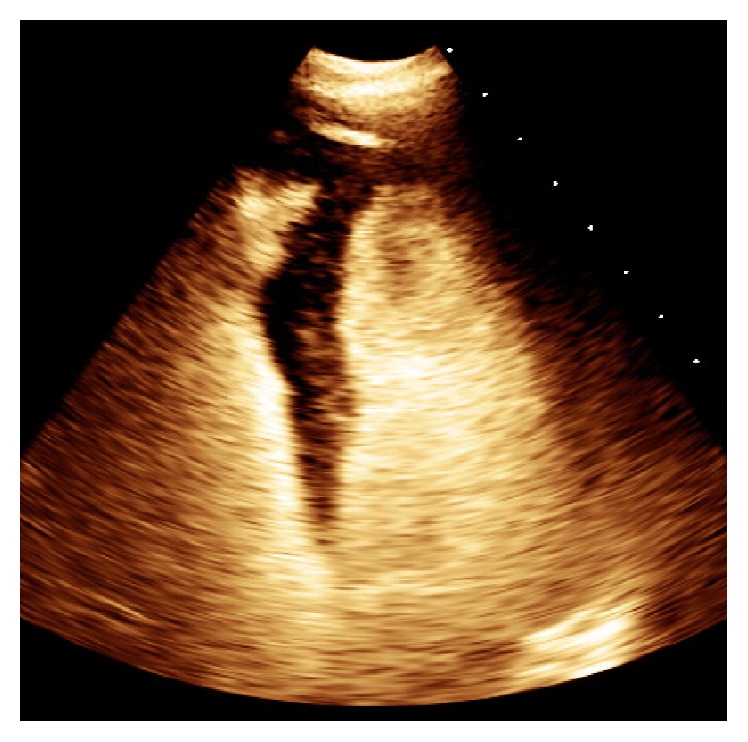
Complete resolution of interventricular septal hematoma at 3 months after PCI. (Video 3 shows follow-up echocardiographic findings and is included as supplementary material online.)
